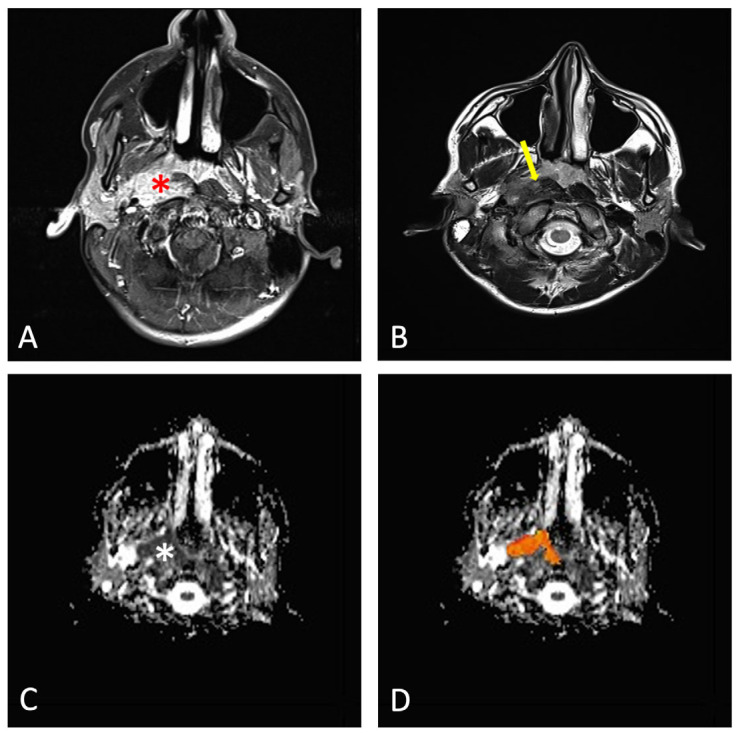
A 13-year-old child with NPC: (A) post-contrast axial T1-weighted imaging showing a large nasopharyngeal mass (red asterisk); (B) axial T2-weighted imaging demonstrating the involvement of the masticatory space (yellow arrow); (C) ADC map showing low signal intensity of the nasopharyngeal mass (white asterisk); (D) ROI was defined by covering as much tumor tissue as possible (shown on the slice with the maximum tumor diameter, orange area).
